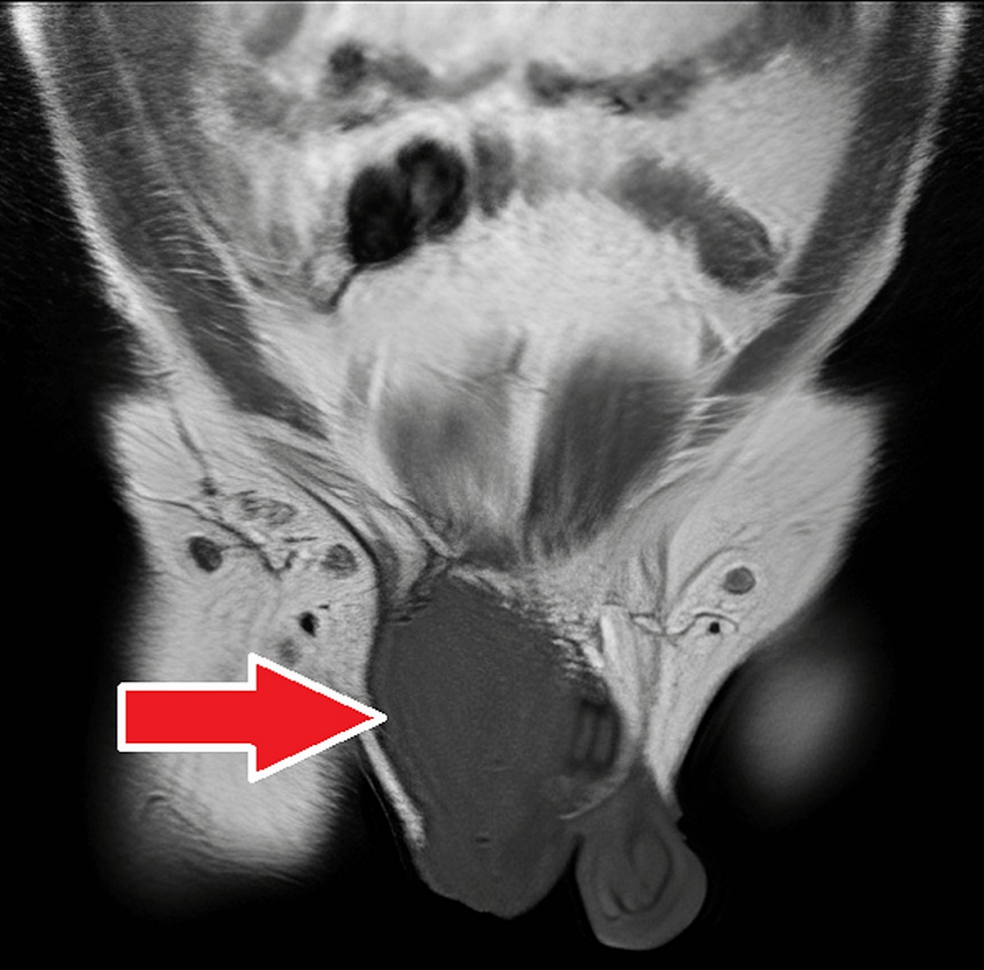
MRI - coronal section of the pelvis The image demonstrates a T1-weighted coronal section of the pelvis that shows diffusely hypointense relatively well-defined mass over the right inguinal region extending into the ipsilateral scrotal sac; contralateral testis and hemiscrotum appear normal MRI: magnetic resonance imaging
